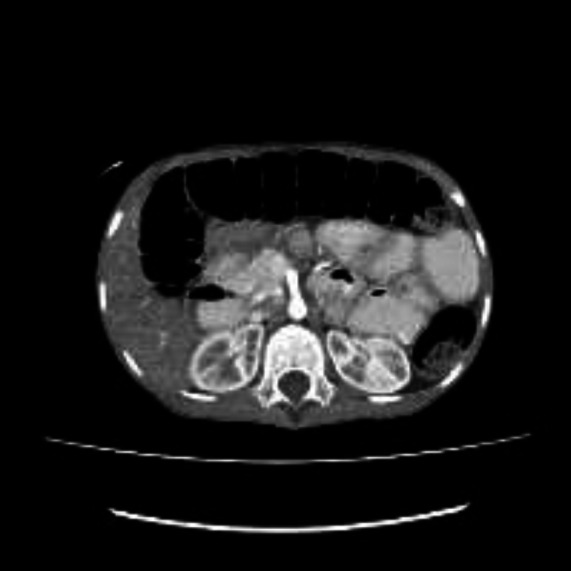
Axial CT at the levels of the kidneys, showing dilated small bowel loops (blue arrows)
